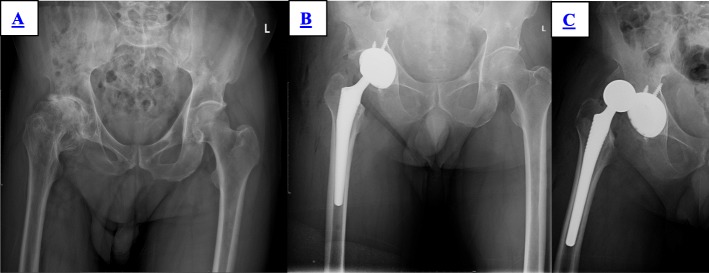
Male, 38 years old. a Preoperative X-ray showed right Crowe I DDH. b Postoperative X-ray. c Anterior dislocation in the 1st day postoperatively
